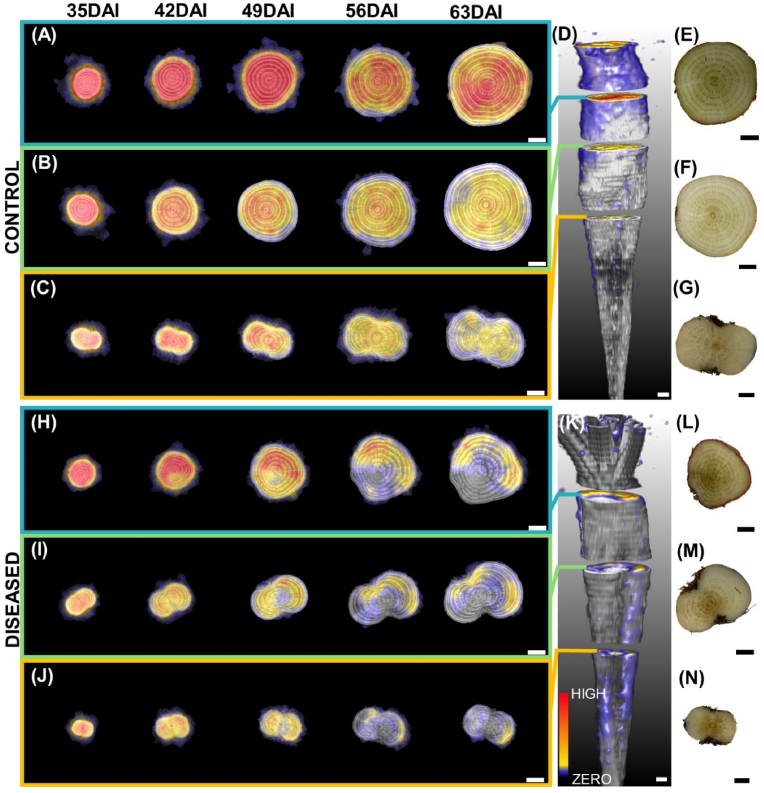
Examples of MRI-PET co-registration showing tracer distribution in the developing taproot as well as RGB images of taproot at destructive analysis for control taproot C1 (A–G) and diseased taproot D1 (H–N). Figure layout is same as in Fig. 4 except for additional PET images overlaid in color. Areas with cooler colors (grey, blue) indicate no or very low ^11^C-tracer activity while areas with warmer colors (yellow and red) indicate high ^11^C- tracer activity. Bars, 0.5 ​cm.
